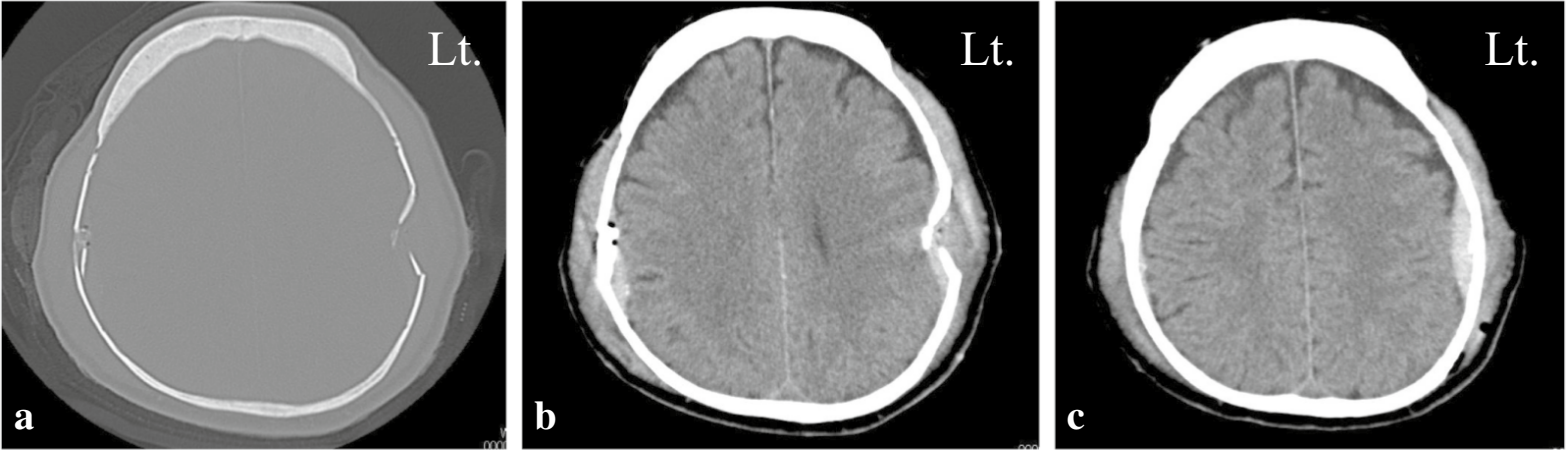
Emergency head computed tomography (CT) showing depressed skull fractures underlying the single-pin sites (a, b) with an associated epidural hematoma on the left (Lt.) side (c)
